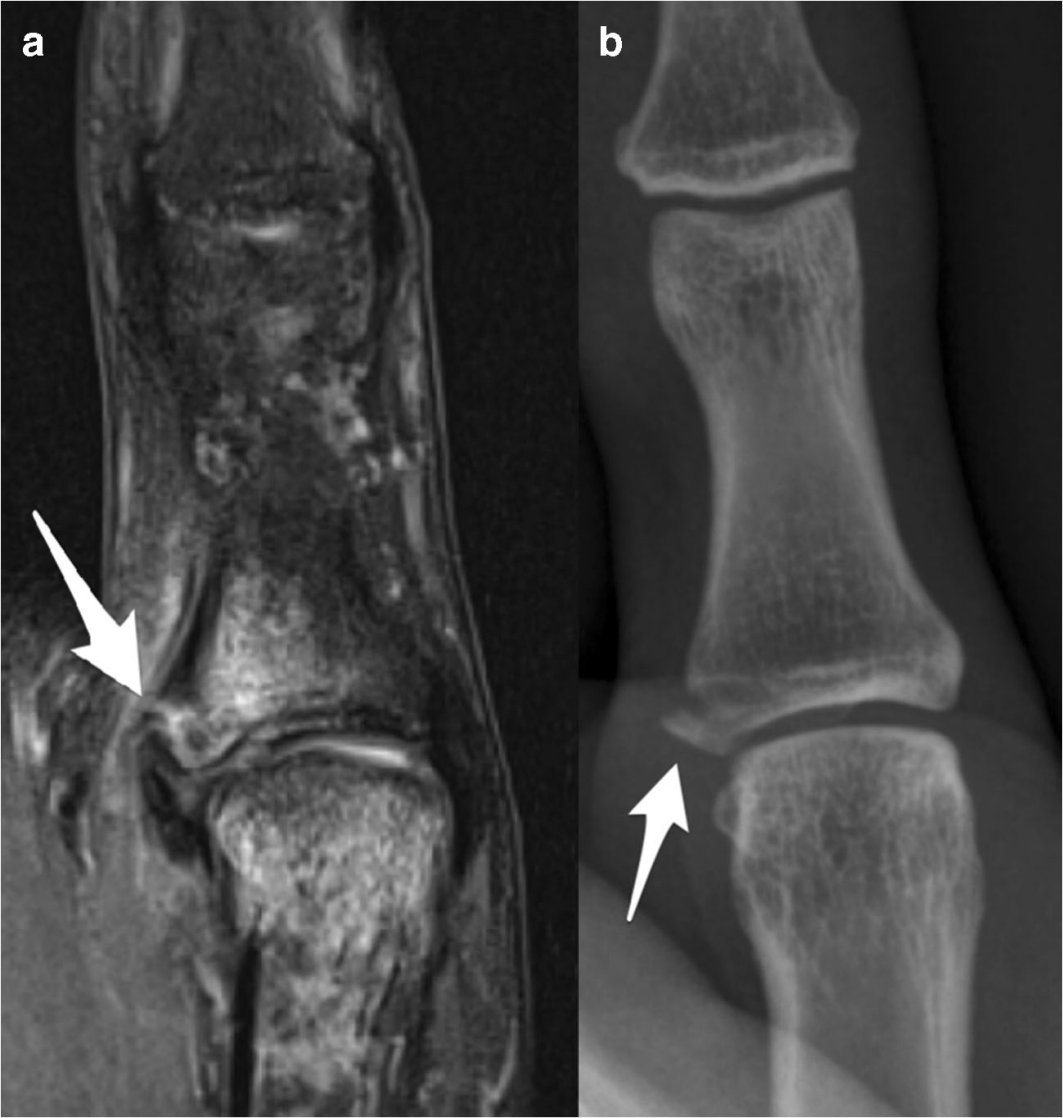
Coronal intermediate-weighted fat-saturated image (a) demonstrates a bony avulsion of the distal insertion of the UCL (arrow in a). The corresponding palmodorsal radiograph (b) shows the minimally displaced fracture at the ulnar base of the proximal phalanx
